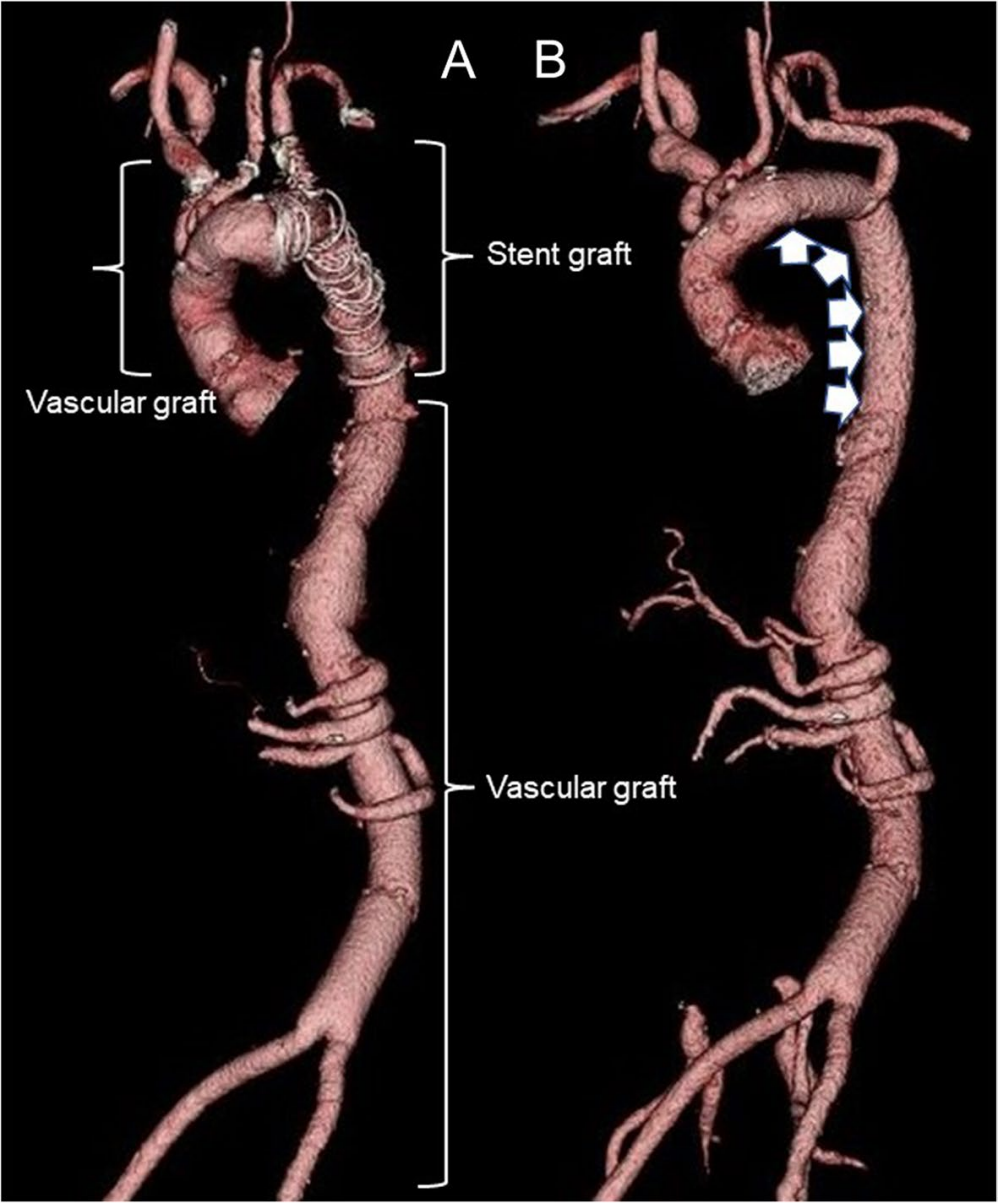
A Three-dimensional contrast-enhanced computed tomography shows the physical relationship between the previous grafts and the endovascular stent graft preoperatively. B Postoperative aortic configuration is demonstrated on three-dimensional contrast-enhanced computed tomography. Arrowheads indicate a newly implanted vascular graft. The left subclavian artery was reconstructed with a side branch after the removal of the previous stent graft
